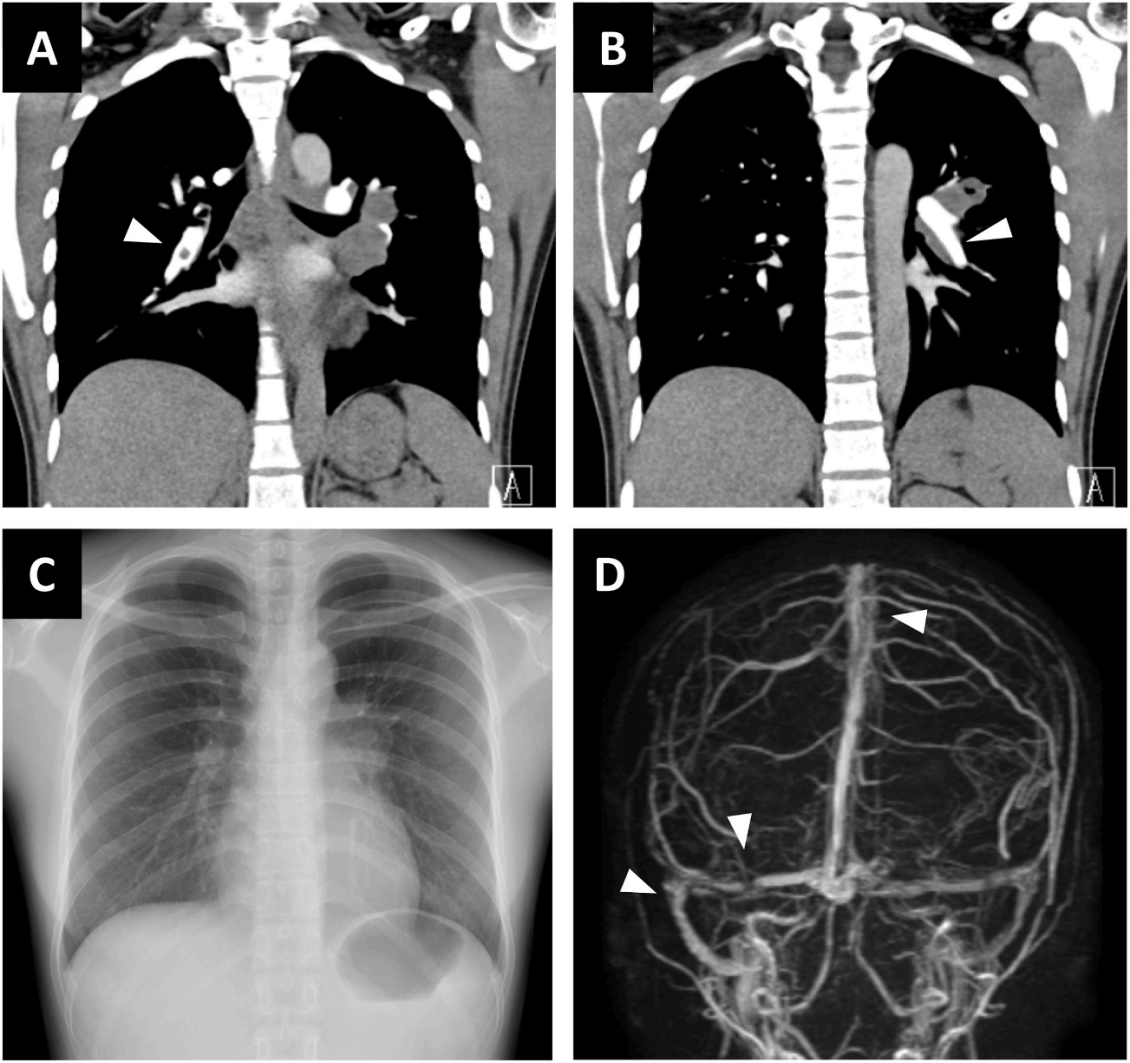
Radiological findings at follow-up A-B. Contrast-enhanced CT of the chest three weeks after the admission. Note that marked amelioration of thromboses in bilateral pulmonary arteries (arrowheads). C. Chest radiography after completion of anti-tuberculous therapy showing shrinkage of the left hilar lymphadenopathy. D. MRV after completion of edoxaban therapy indicating recovery of the venous blood flow in the right cerebral venous sinuses (arrows).
